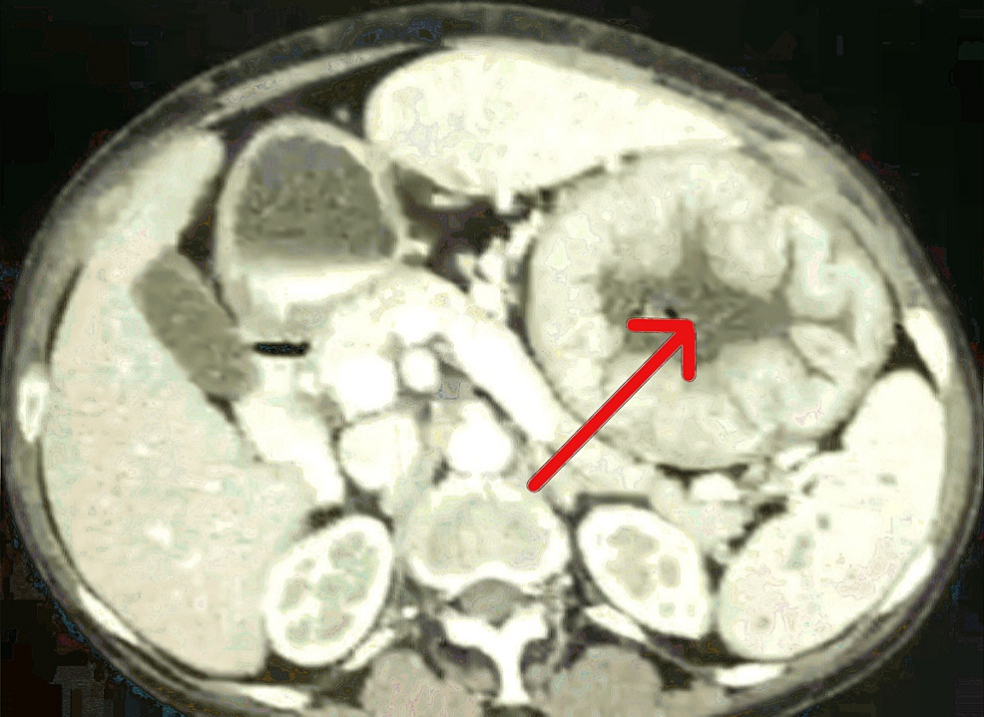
Contrast-enhanced CT of the abdomen: aneurysmal dilatation and circumferential asymmetric thickening of the stomach CT: computed tomography
